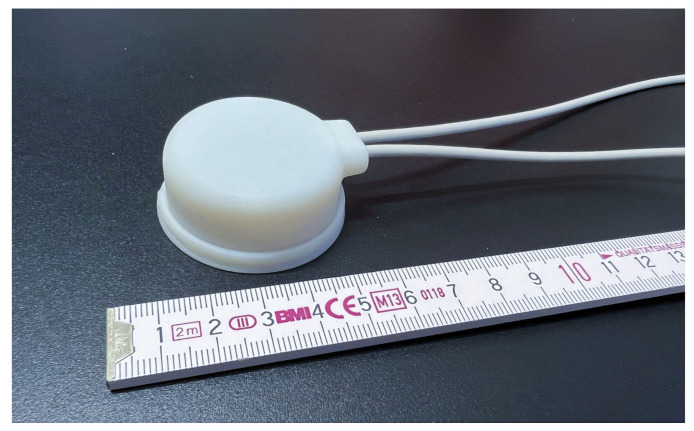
3D-printed ultrasound transducer mockup used in this study.
